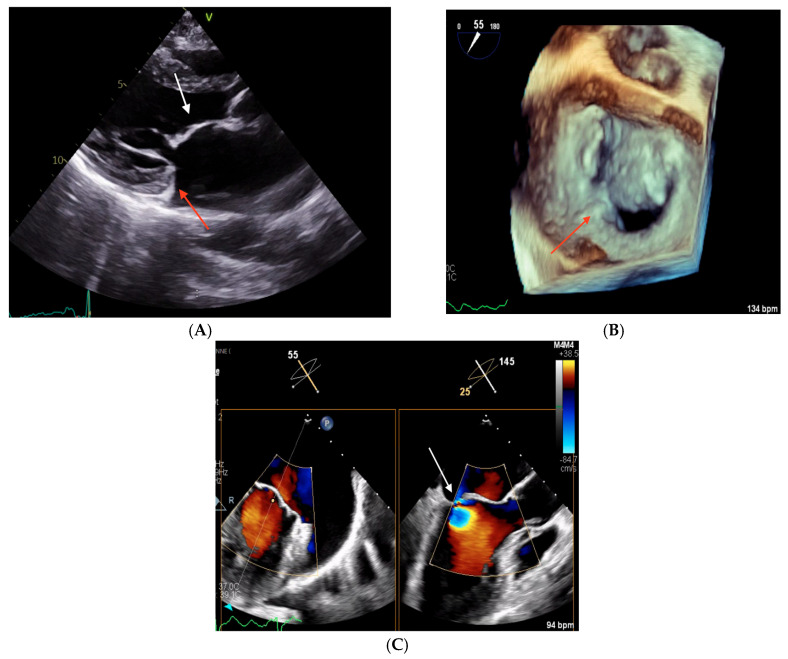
Echocardiographic images obtained in Clinical Case 2. (A) TTE parasternal long-axis view showing a thickened AML (white arrow) and an hypoplastic PML (red arrow). (B) 3D TEE “en face” view of the mitral valve showing severe PML hypoplasia with only a small P1 scallop (red arrow). (C) 2D TEE 55° mid-esophageal view (left panel) and the orthogonal plane crossing A2/P2, where the origin of the MR jet is located (right panel, white arrow).
